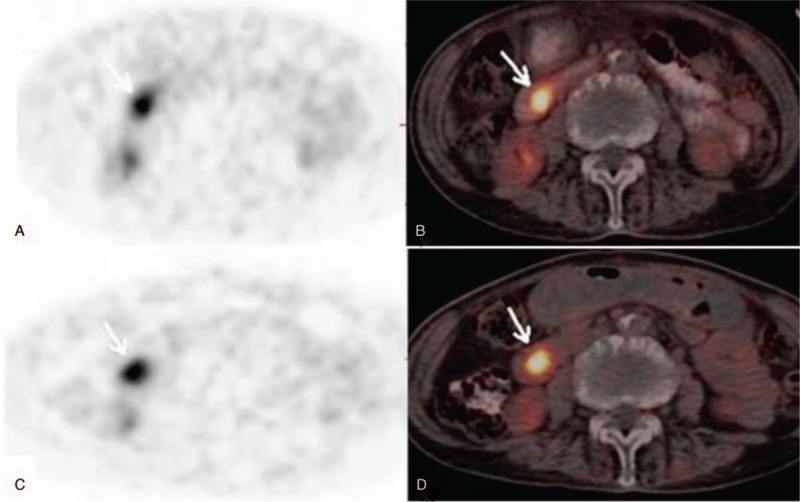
A malignant duodenal lesion (adenocarcinoma) in a 72-year-old female shows significant uptake on early (A, B) and delayed (C, D) ^18^F-FDG PET/CT imaging. The SUV_max_ increases from 7.1 on the early stage to 7.3 on the delayed stage. ^18^F-FDG = fluorine-18 fluorodeoxyglucose, PET/CT = positron emission tomography/ computed tomography, SUV_max_ = maximum standardized uptake value.
